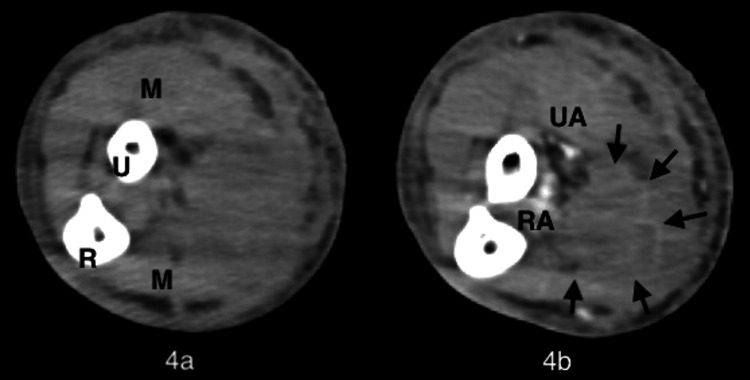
Computed tomography (CT) of the right upper extremity (a) Plane CT; (b) contrast-enhanced CT. The arterial and muscular contrast effects are good. The area surrounded by arrows shows poor internal contrast and partial external contrast, suggesting a hematoma. Arrows, hematoma; M, muscles; R, radius; RA, radial artery; U, ulna; UA, ulnar artery.
